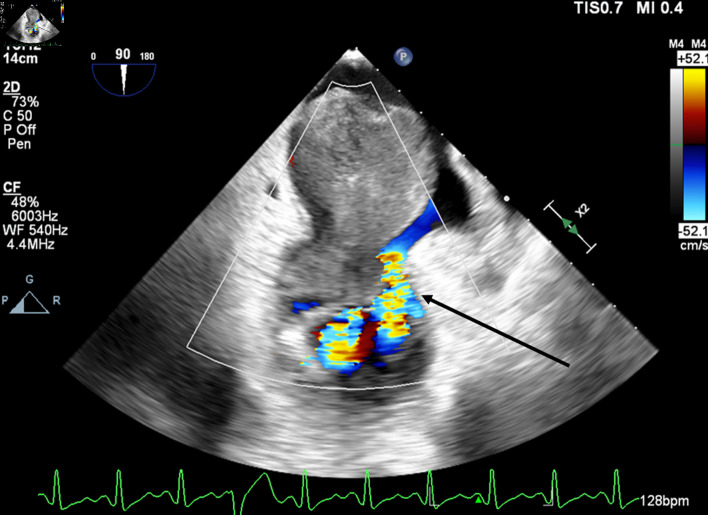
Mid-esophageal two-chamber view with color flow Doppler demonstrating turbulent flow around the mass through the mitral valve during diastole. The arrow indicates turbulent flow through the mitral valve.
